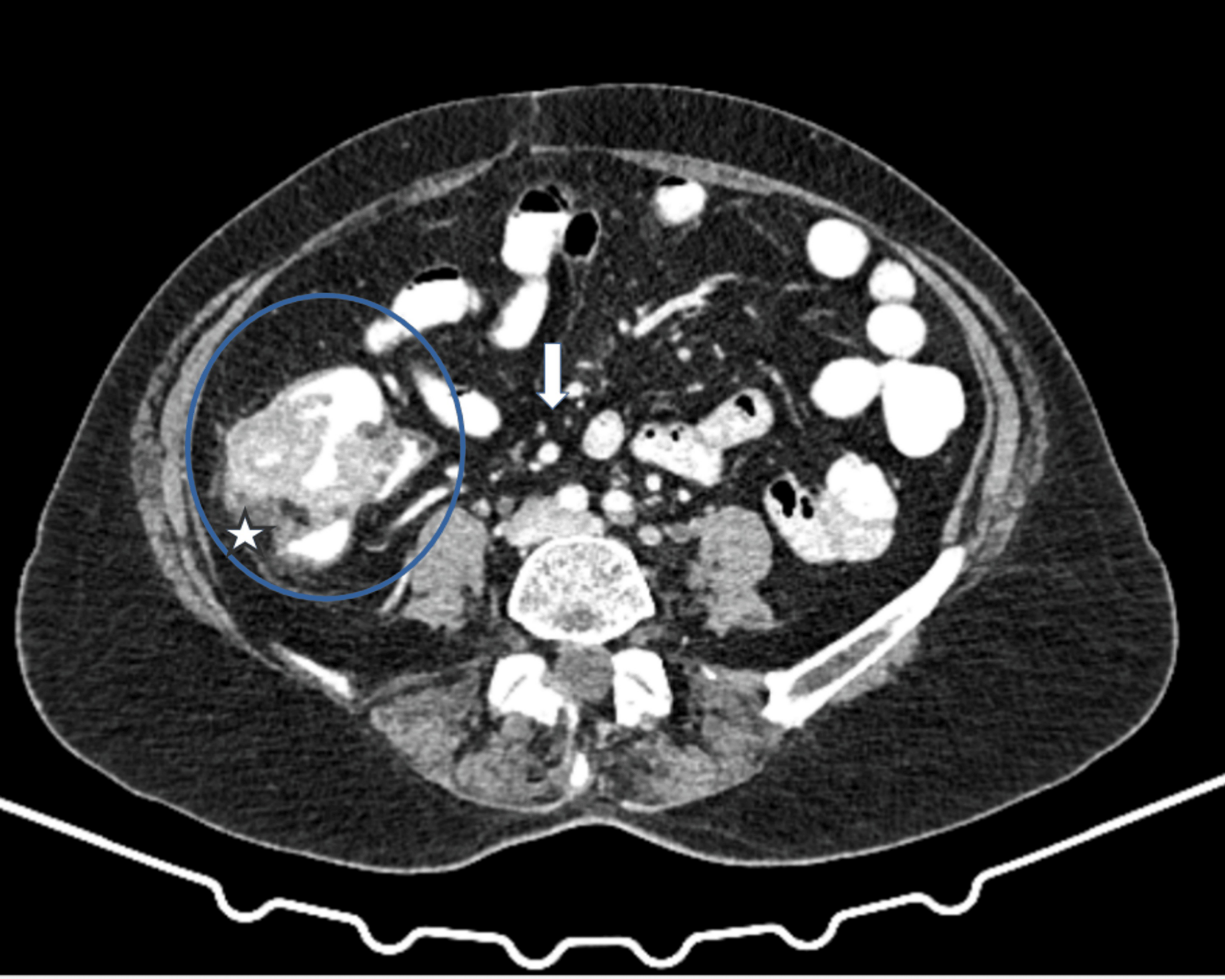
A CT scan of the abdomen with intravenous contrast (axial view) demonstrated cecal dilatation with associated fecal impaction (blue circle). The pericolic fat, particularly in the post-cecal region, showed stranding suggestive of localized inflammation (white asterisk). Additionally, a few enlarged lymph nodes were visible, raising suspicion for reactive lymphadenopathy (white arrow).
